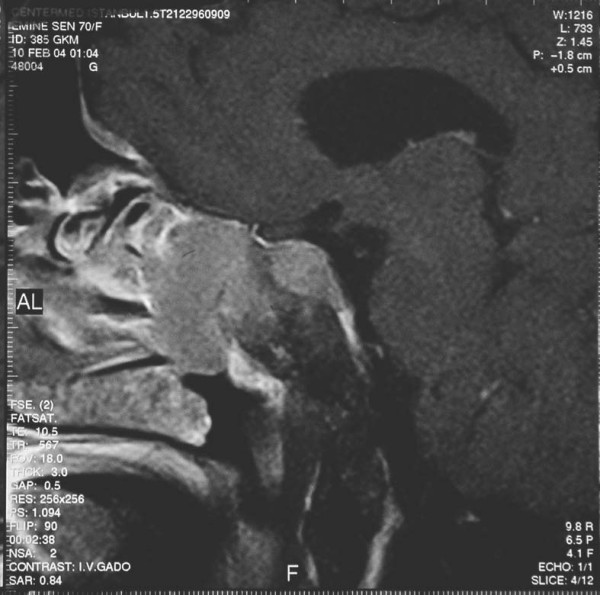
Sagittal pituitary magnetic resonance imaging T1W1 with contrast agent applied.
